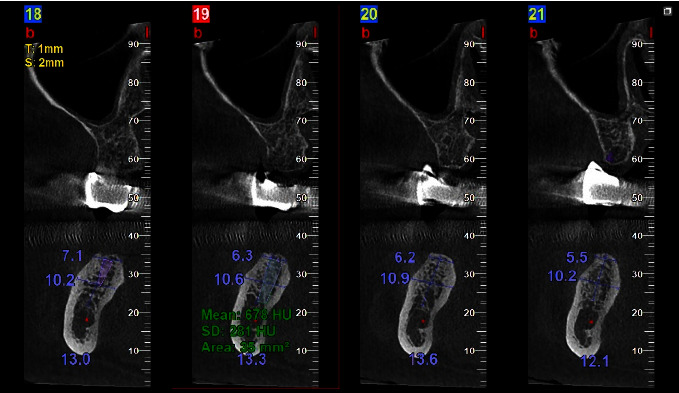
CBCT's coronal view showing a virtual dental implant with the estimated bone density inside it.
